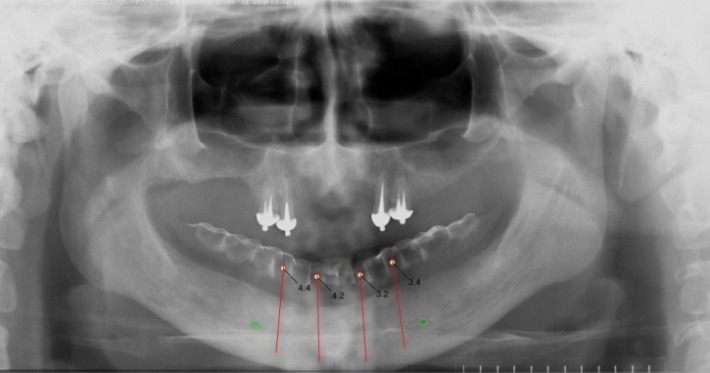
The placement of the mini implants using the X-ray guide
